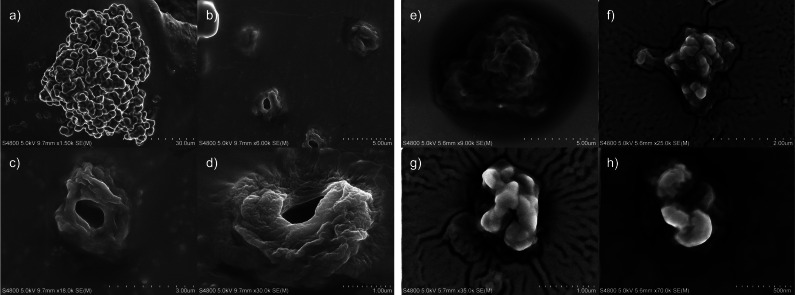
SEM analysis of cellulose microparticles after regeneration from [P_4441_][OAc]:GVL (a–d) and washing away of residual [P_4441_][OAc] using acetone and ultrasonication (e–f): a) microparticle cluster, b–d) separated microparticles retained on the support after flushing with nitrogen, e‐h) aggregated and fully regenerated microparticles of different sizes after washing away the [P_4441_][OAc].
